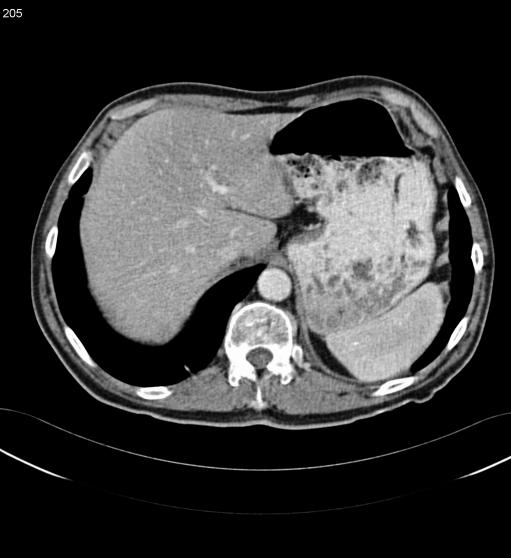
Post-Treatment CT Showing Almost Complete Resolution of the Abnormal Thickening of Gastro-Oesophageal Junction and Stomach Wall
